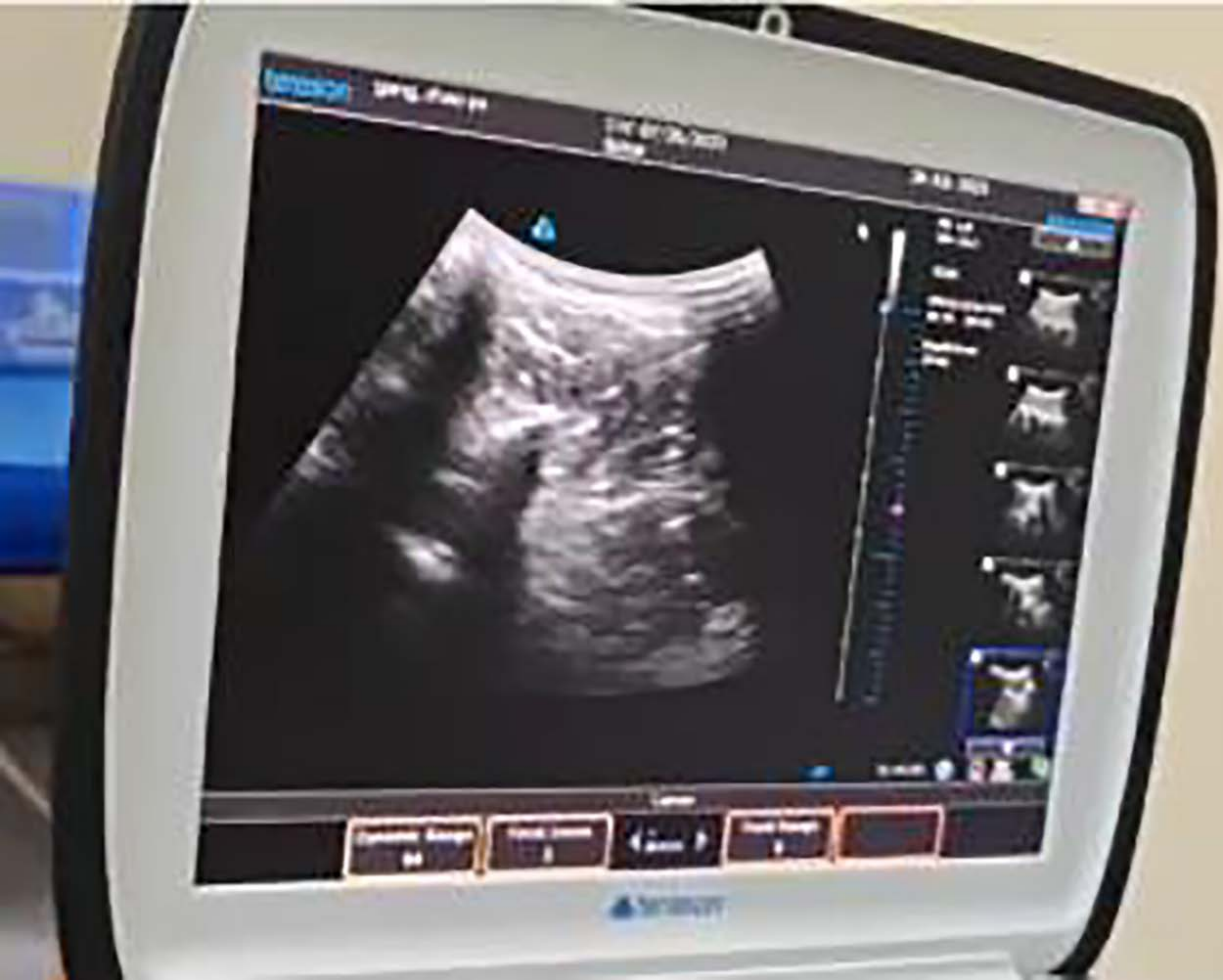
Ultrasound-guided trigger point needling.
